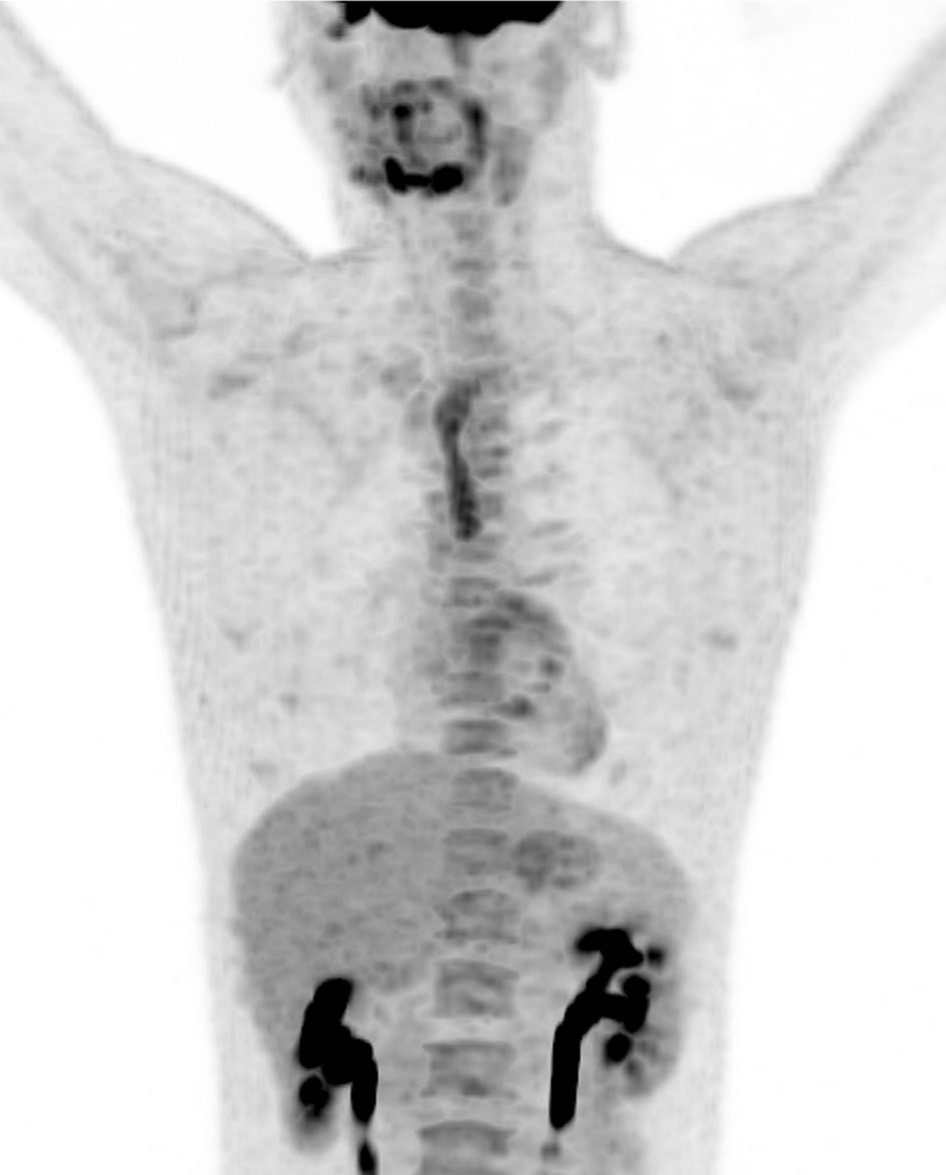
Maximum intensity projection (MIP) of FDG PET/CTA of same patient, physiologic, high activity of FDG in the thymus
